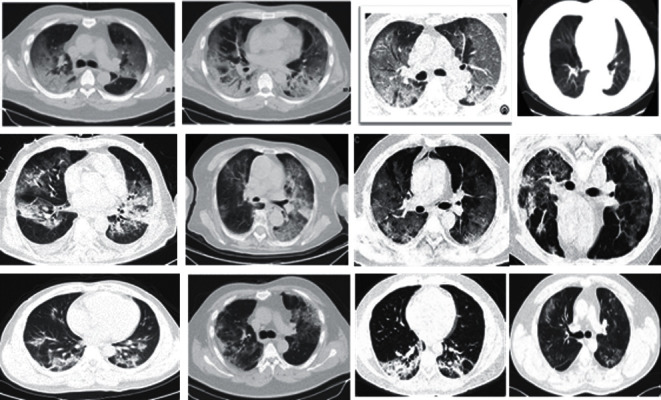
COVID-19 CT lung scan cases.
